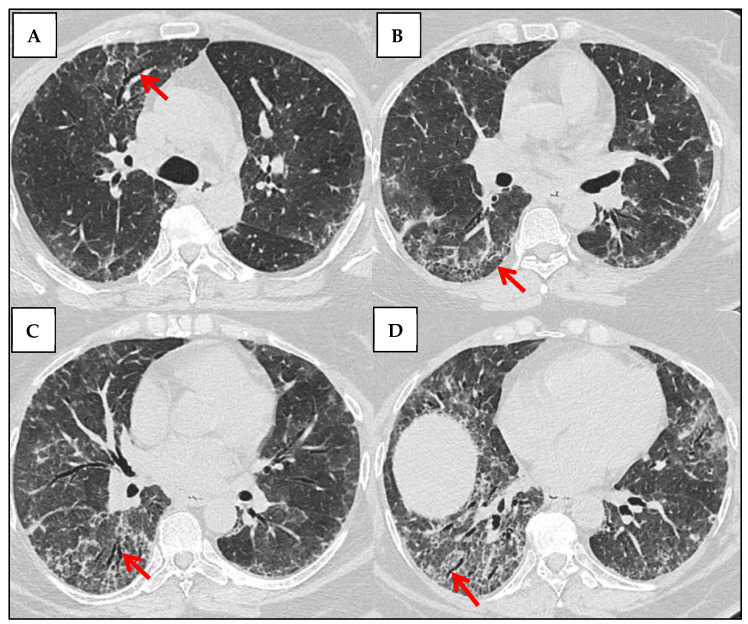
HRCT (2019) Demonstrating a Fibrotic NSIP Pattern in a Patient with Primary Sjögren’s Syndrome. A 49-year-old female with a 30 pack-year smoking history presented in February 2019 with progressive exertional dyspnea (mMRC grade 3) and significant resting hypoxemia (SpO_2_ 80% on room air). Serologic evaluation revealed positive anti-SSA (Ro) and anti-SSB (La) antibodies, accompanied by clinical manifestations of xerophthalmia and xerostomia, establishing the diagnosis of primary Sjögren’s syndrome. The axial HRCT images from 2019 demonstrate the characteristic radiological pattern of fibrotic NSIP. The images show bilateral, symmetric reticular opacities and ground-glass changes predominantly in the lower lung zones. There is clear subpleural sparing (B), visible as a thin rim of relatively preserved lung immediately beneath the pleura, which is a distinguishing feature of NSIP (red arrow). Architectural distortion and lower lobe volume loss are present, but there is no evidence of honeycombing. In addition to these findings, there is clear evidence of traction bronchiolectasis. This is seen as irregular, dilated bronchioles within areas of fibrotic lung parenchyma, reflecting traction on the small airways (A,C,D–red arrows).
